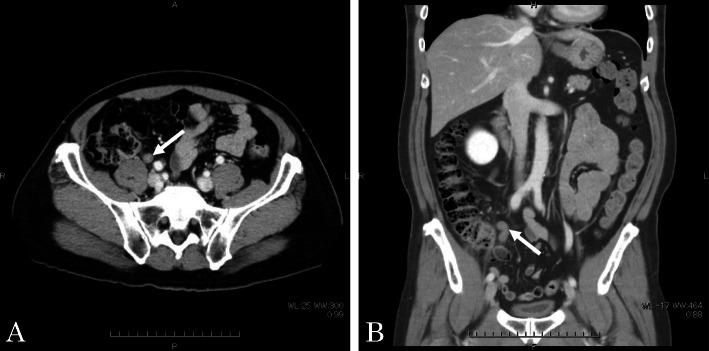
Abdominal computed tomography (a: axial, b: sagittal). Appendix is slightly swollen (arrow) without obvious neoplastic tumors or lymphadenopathy
